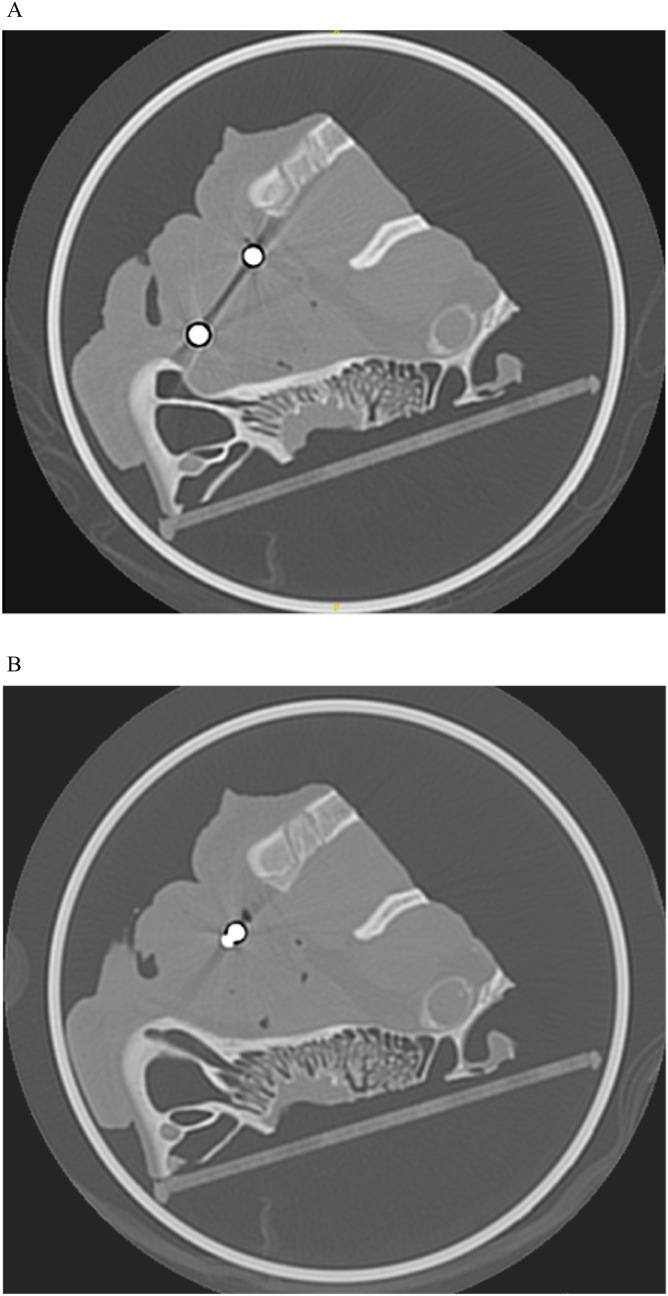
The pre- (a) and post-MRI (b) CT scans of two intravitreal 4-mm ferromagnetic foreign bodies show the post-MRI displacement including an attractive effect.
